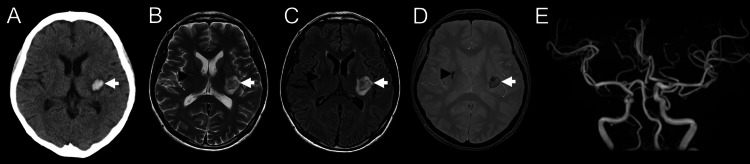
Neuroimaging findings of putaminal hemorrhage. (A) Non-contrast head computed tomography on admission showing a small acute hemorrhage in the left putamen, measuring approximately 13 mm in maximum diameter (white arrow). (B, C) Axial T2-weighted and fluid-attenuated inversion recovery magnetic resonance images on hospital day 2 demonstrating the left putaminal hemorrhage with surrounding edema extending toward the thalamic region (white arrows). This peri-putaminal lesion was located near the internal capsule and thalamocortical projection area, where transient involvement of adjacent subcortical gustatory pathways was considered possible. (D) T2*-weighted imaging showing a hypointense lesion corresponding to the acute left putaminal hemorrhage (white arrow) and an old right putaminal hemorrhagic lesion suggestive of a previous hemorrhagic scar (black arrowhead). (E) Magnetic resonance angiography showing no vascular malformation, aneurysm, or major arterial stenosis.
